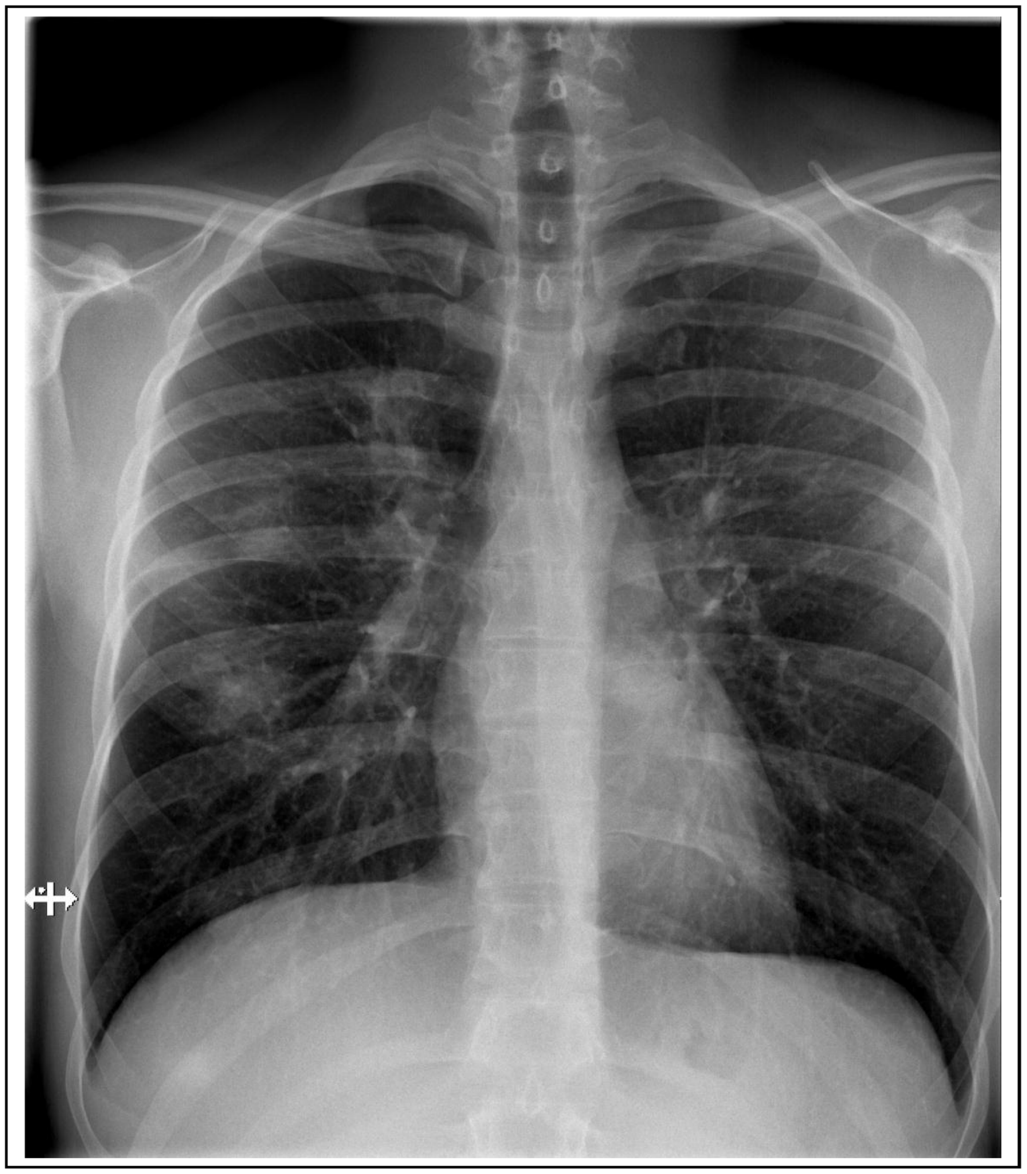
Chest X-ray showing patchy infiltrates in both lungs.
